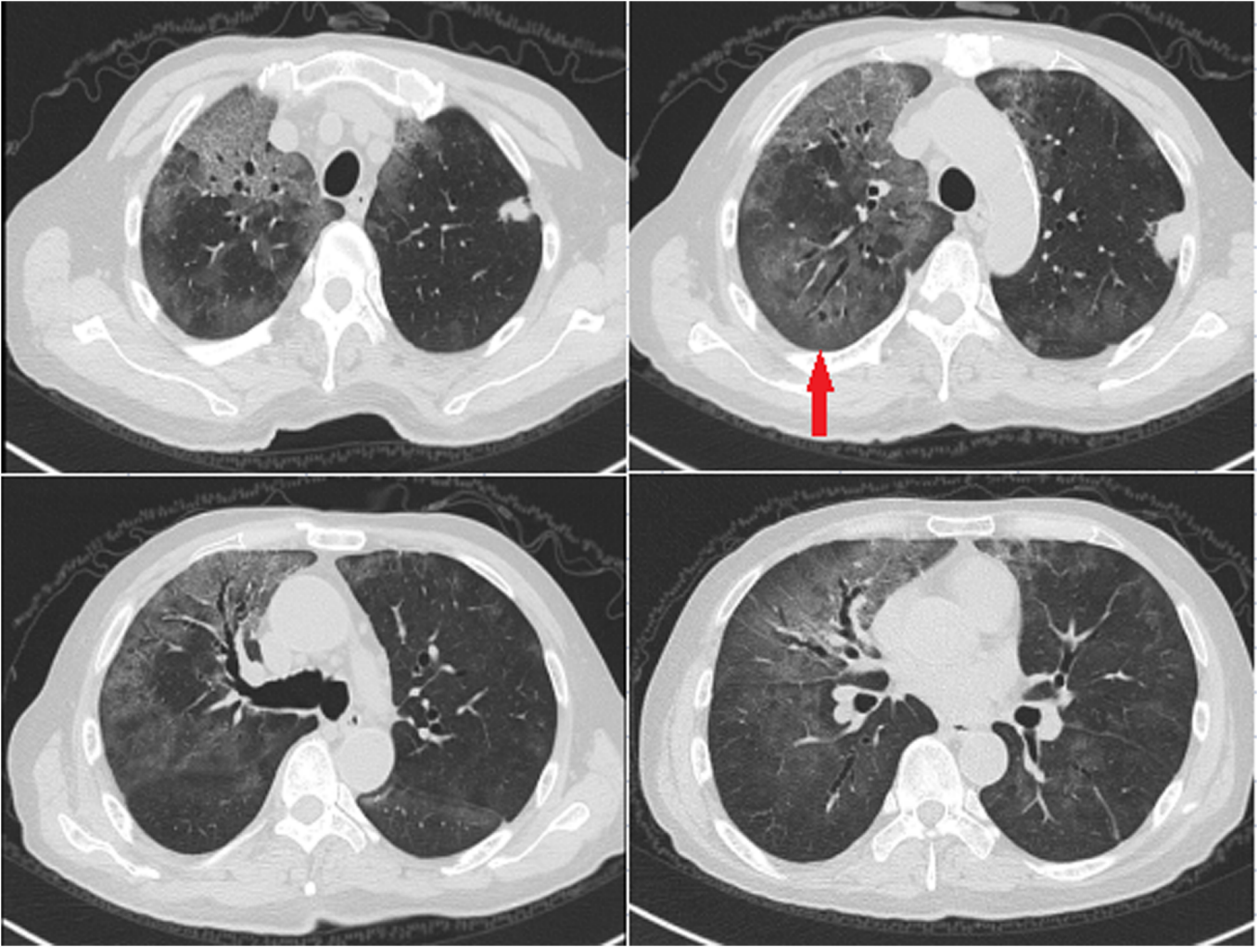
Repeated chest CT showing persistently progressed ground-glass opacities in bilateral lungs. Transbronchial lung biopsy was performed in the right upper lung (red arrow)
